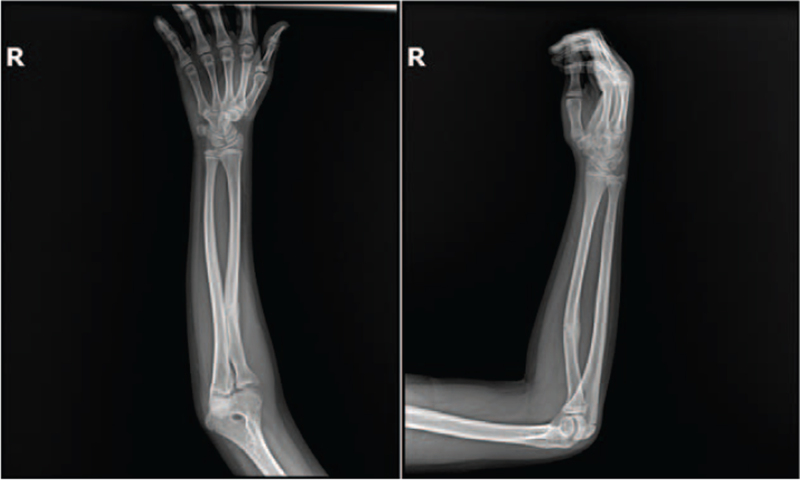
Radiographs of the right forearm performed at the time of injury. Anteroposterior and lateral radiographs showing linear fracture without displacement of the radius at the proximal third, but a radial fracture with an anterior convex deformity of 8°. No radial head dislocation was observed.
